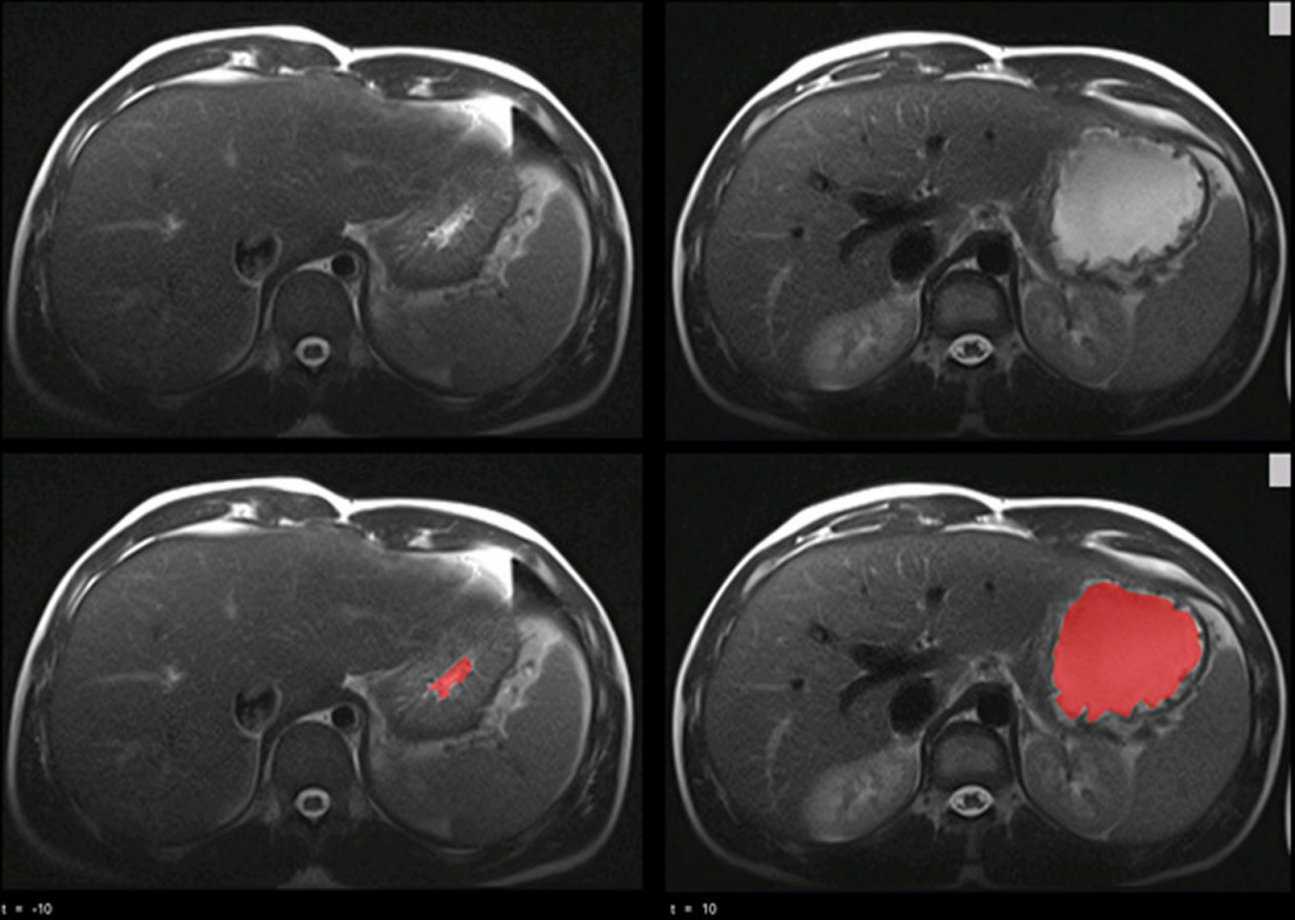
Example of volume segmentation in a T2‐weighted spin‐echo magnetic resonance image taken at the height of the liver, showing pre‐ingestion on the left and at 10 min post‐digestion on the right. In red, the segmented volume. © 2018 The Authors. Neurogastroenterology & Motility Published by John Wiley & Sons Ltd
